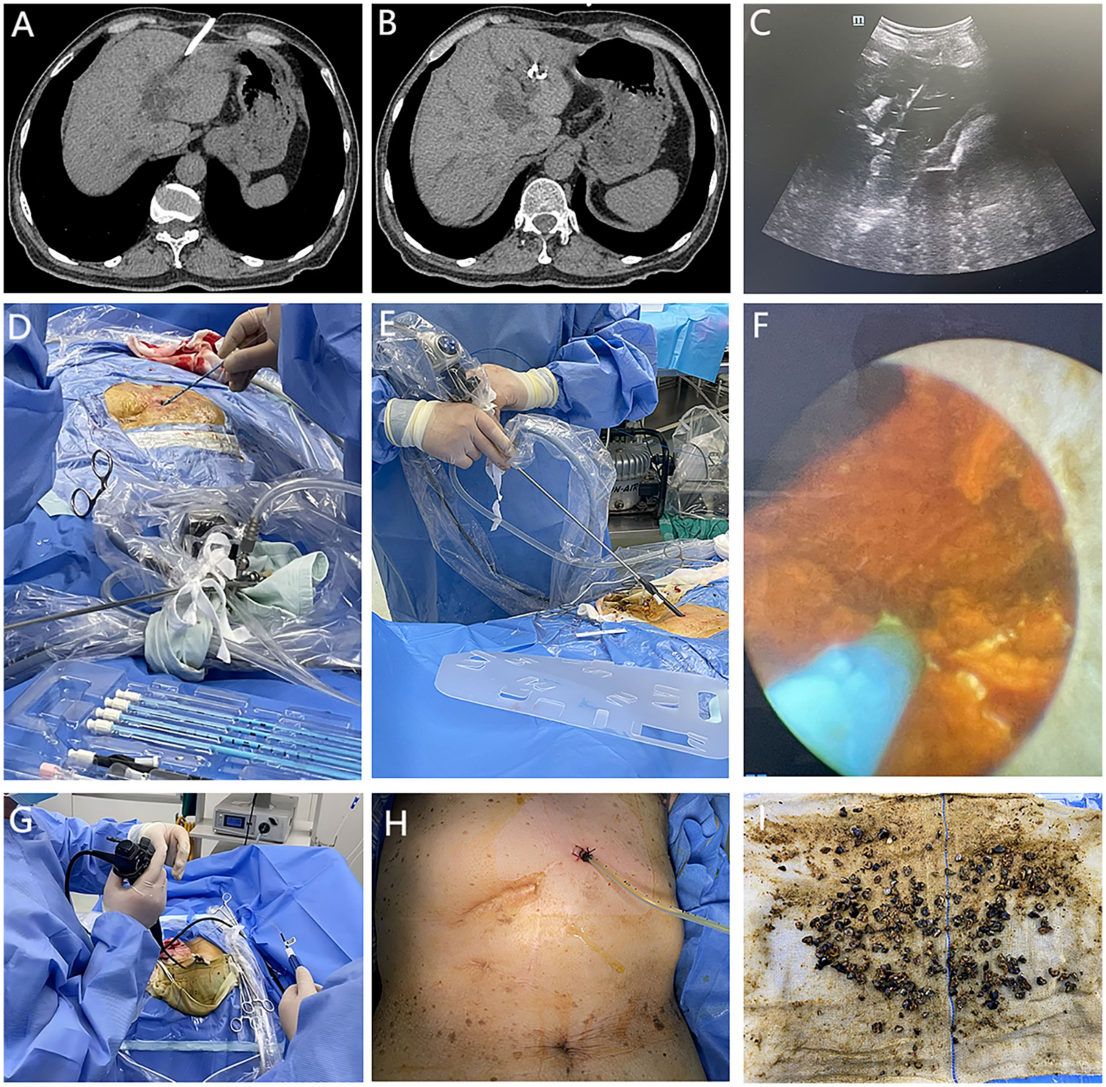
(A,B) Non-contrast CT images showing the positional relationship between the PTCD catheter and intrahepatic bile ducts; (C–I) Sequential visualization of the procedural steps for PTCSL.
